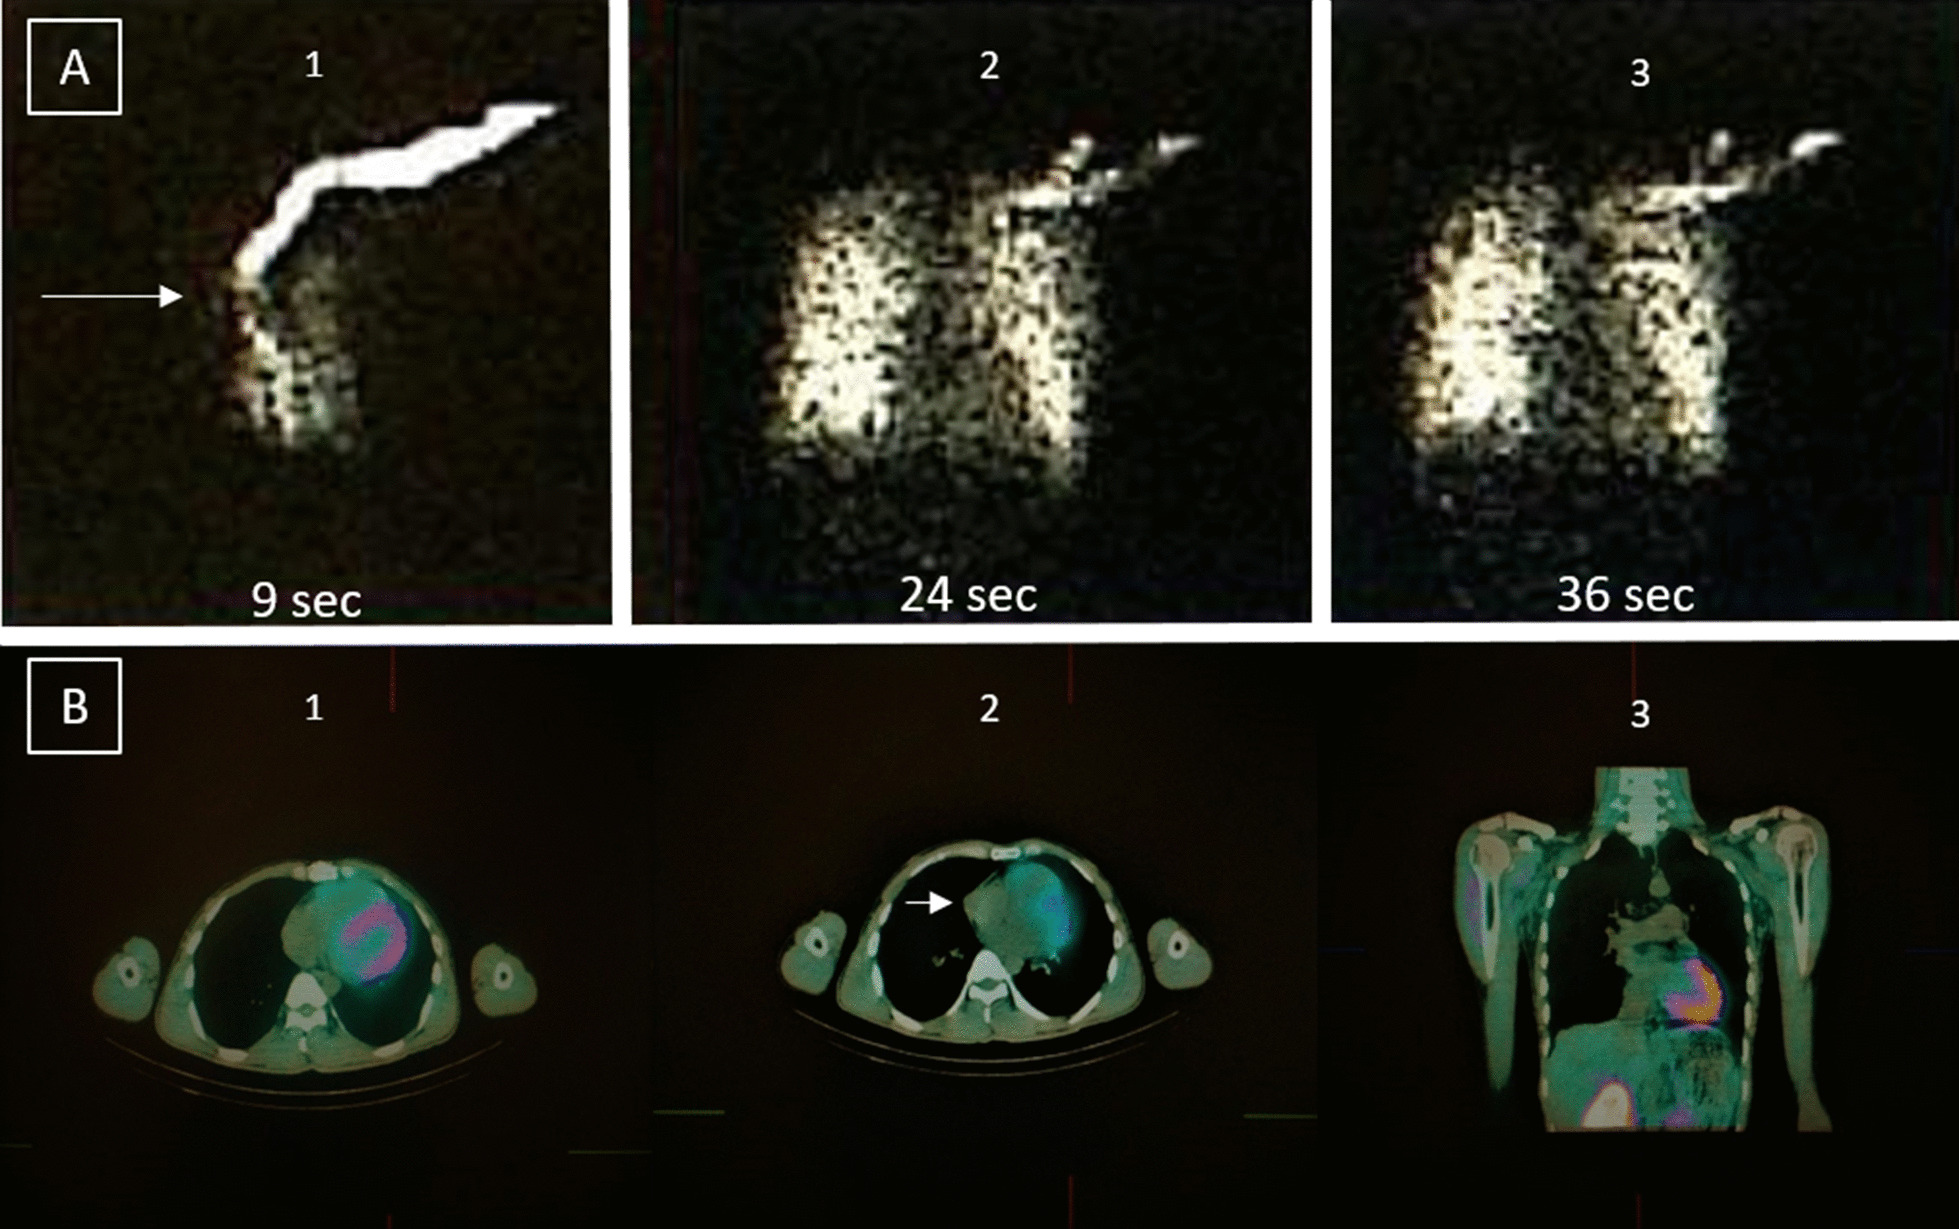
Nuclear Imaging. SPECT/CT with TC99m -MAA (a) and Sestamibi (b), filling defect (white arrow) in radioactivity in SVC before entering RA (a1); No radioactivity traced in the cardiac chamber (if there was intracardiac mass should be detected from a2). Physiologic radioactivity uptake of the myocardium (b), with no increased radioactivity uptake in pericardial mass near RA (b2) and RV (b3)
